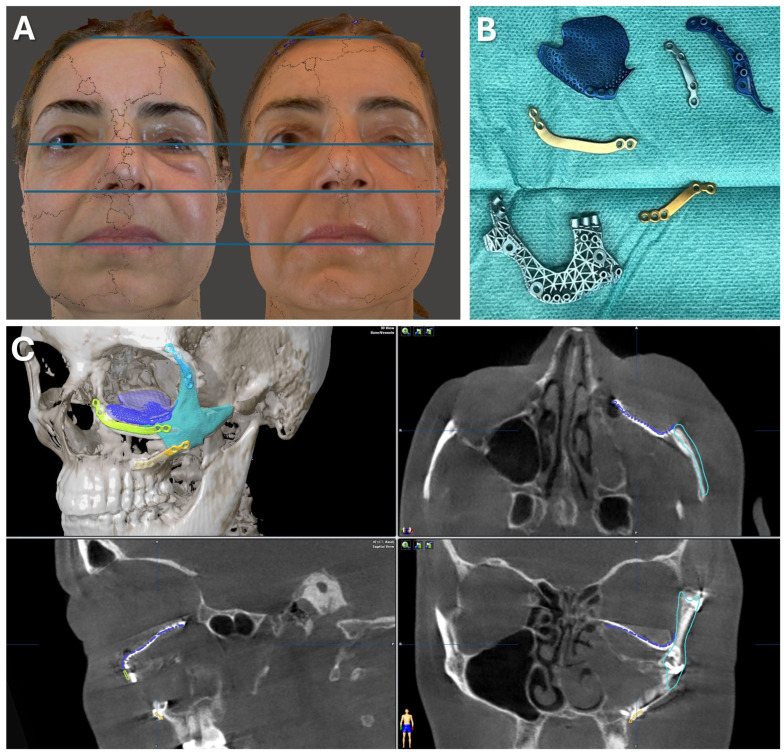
Case 1—Secondary repair of misplaced zygoma and orbital floor reconstruction. (A) Comparison of pre- and postoperative face scans shows that the asymmetry of the eyelids due to the hypoglobus and enophthalmos of the atrophic eyeball could be corrected. (B) Laser-sintered, color-coded patient-specific implants with corresponding drilling and cutting guides. (C) Although the guides could not be correctly positioned due to pseudarthrosis, the reconstruction results regarding the repositioned zygoma and the orbital implant placements were successful with accurate positioning due to intraoperative surgical navigation, which was proved by post-surgical analysis via the matching of the postoperative CBCT scan to the planning data shown in multi-planar view.
